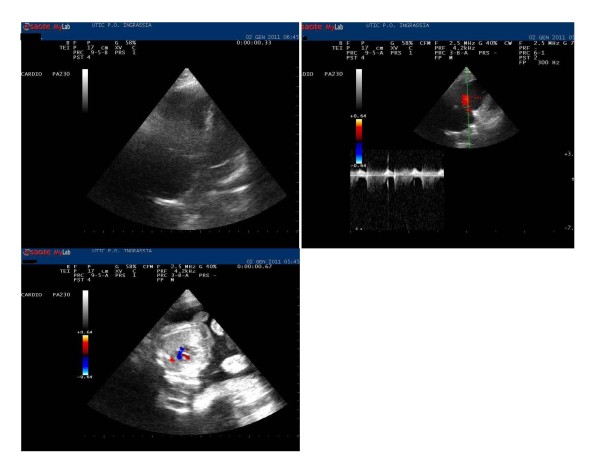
On admission: right ventricular dysfunction and fetus echocardiogram 4 h after thrombolysis.
